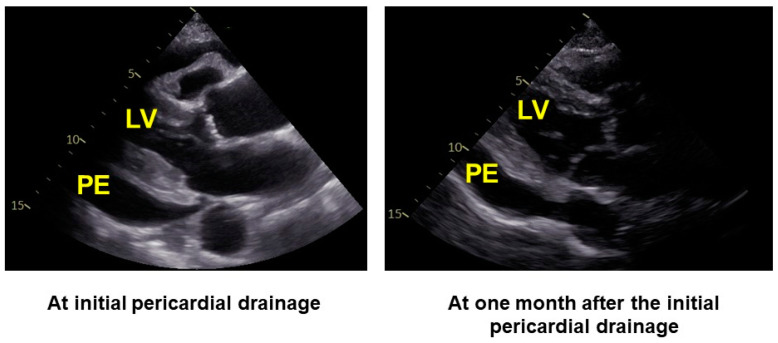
A representative patient with LVDD < 42 mm at baseline who experienced recurrent pericardial effusion. The patient was a 91-year-old man with a history of hypertension. He was admitted to our outpatient clinic complaining of bilateral leg edema and presenting 2187 pg/mL of serum N-terminal pro B-type natriuretic peptide level. A transthoracic echocardiography showed a huge amount of idiopathic pericardial effusion with stable hemodynamics. We underwent scheduled drainage for pericardial effusion without any complications. One month after the index discharge, he was again admitted to our outpatient clinic with recurrent pericardial effusion. LVDD was 33 mm on the first admission and 28 mm on the second admission. LV, left ventricle; PE, pericardial effusion.
